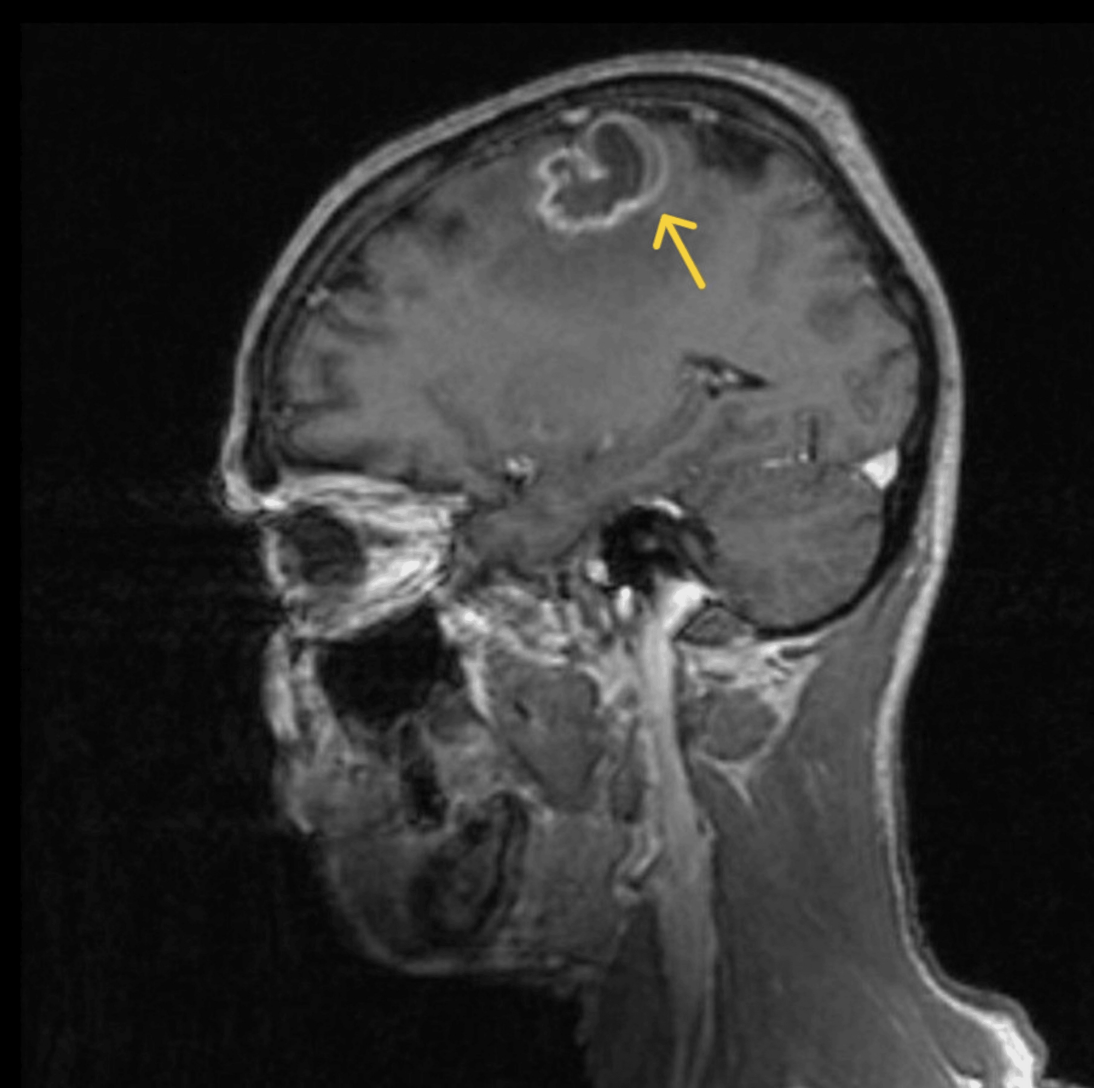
Contrast-enhanced sagittal MRI showing a ring-enhancing lesion with surrounding edema in the left frontal lobe, consistent with a tuberculoma, as indicated by the arrow.
